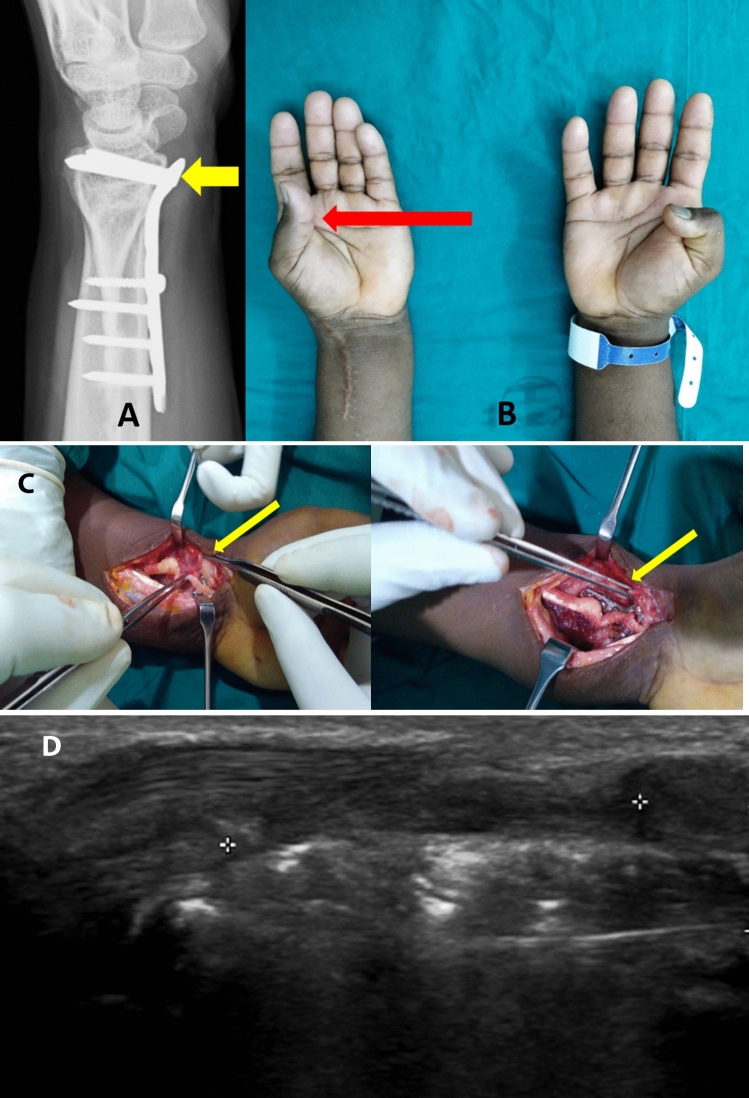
a Lateral radiographs of the wrist with grade 2 plate position as pointed by the yellow pointer. b Clinical photographs showing the inability to flex the interphalangeal joint of the thumb on left side suggestive of flexor pollicis longus injury (red pointer). c Intra-operative photographs showing the thinned out FPL tendon with attrition and close proximity to the distal edge of the plate as shown by the yellow pointer. d USG image showing the thinned out and attenuated FPL tendon with FPL tear. Gap between the torn ends shown by the white markers (+) on the image
